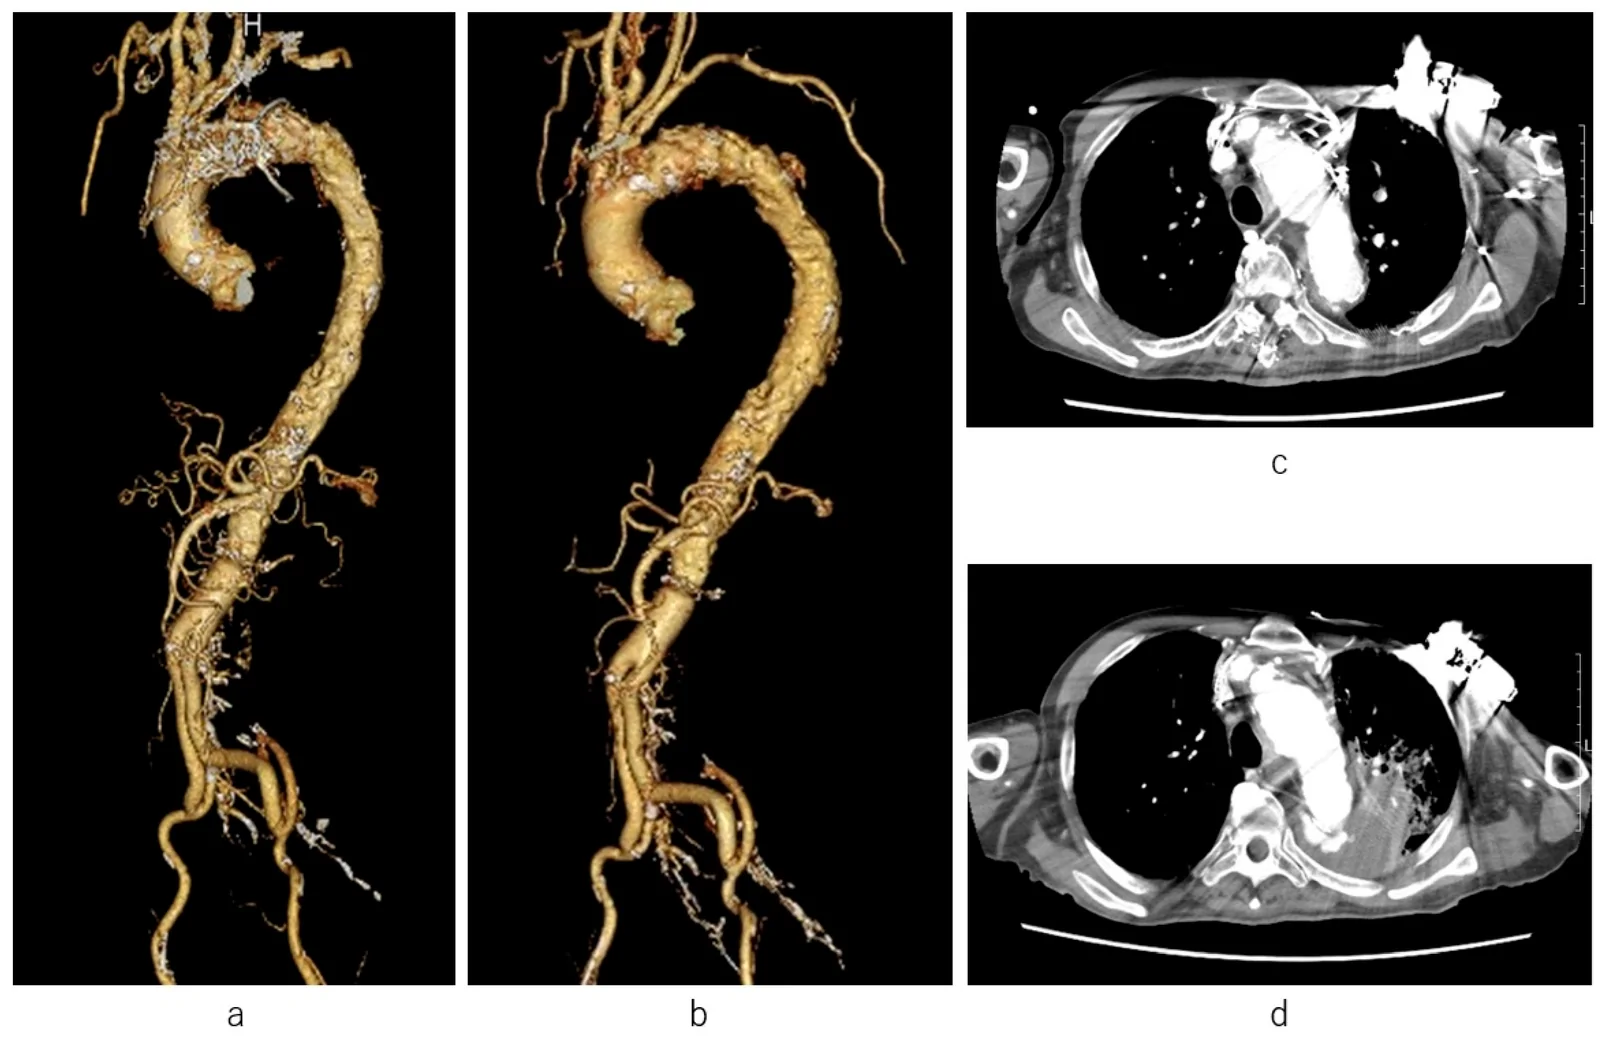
Contrast-enhanced computed tomography findings before and after the onset of hemoptysis. (a,c) Initial computed tomography showed acute type B aortic dissection with a small ulcer-like projection and no pulmonary infiltrative shadow in the left upper lobe. (b,d) After the onset of hemoptysis, the ulcer-like projection enlarged, with contrast inflow into the thrombosed false lumen. An infiltrative shadow appeared in the left upper lobe, suggesting pulmonary hemorrhage due to an aortobronchial fistula involving the peripheral bronchus of the left upper lobe.
